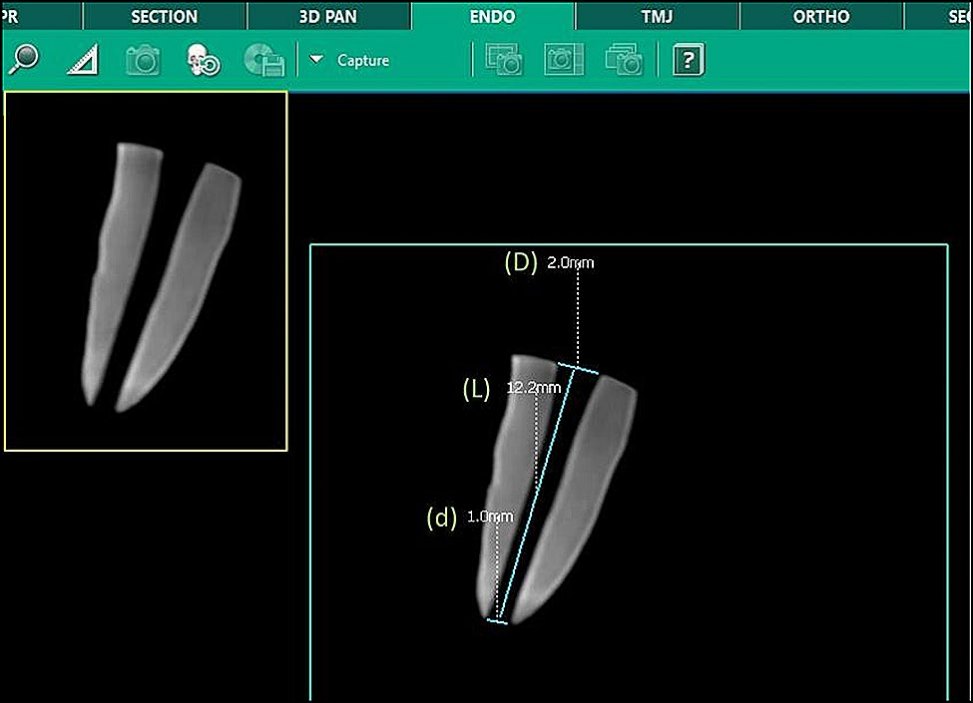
Post-preparation CBCT showing Length (L), Apical Diameter (d), and Coronal Diameter (D) of the root canal used for canal space volume (V_(Canal)_) determination
